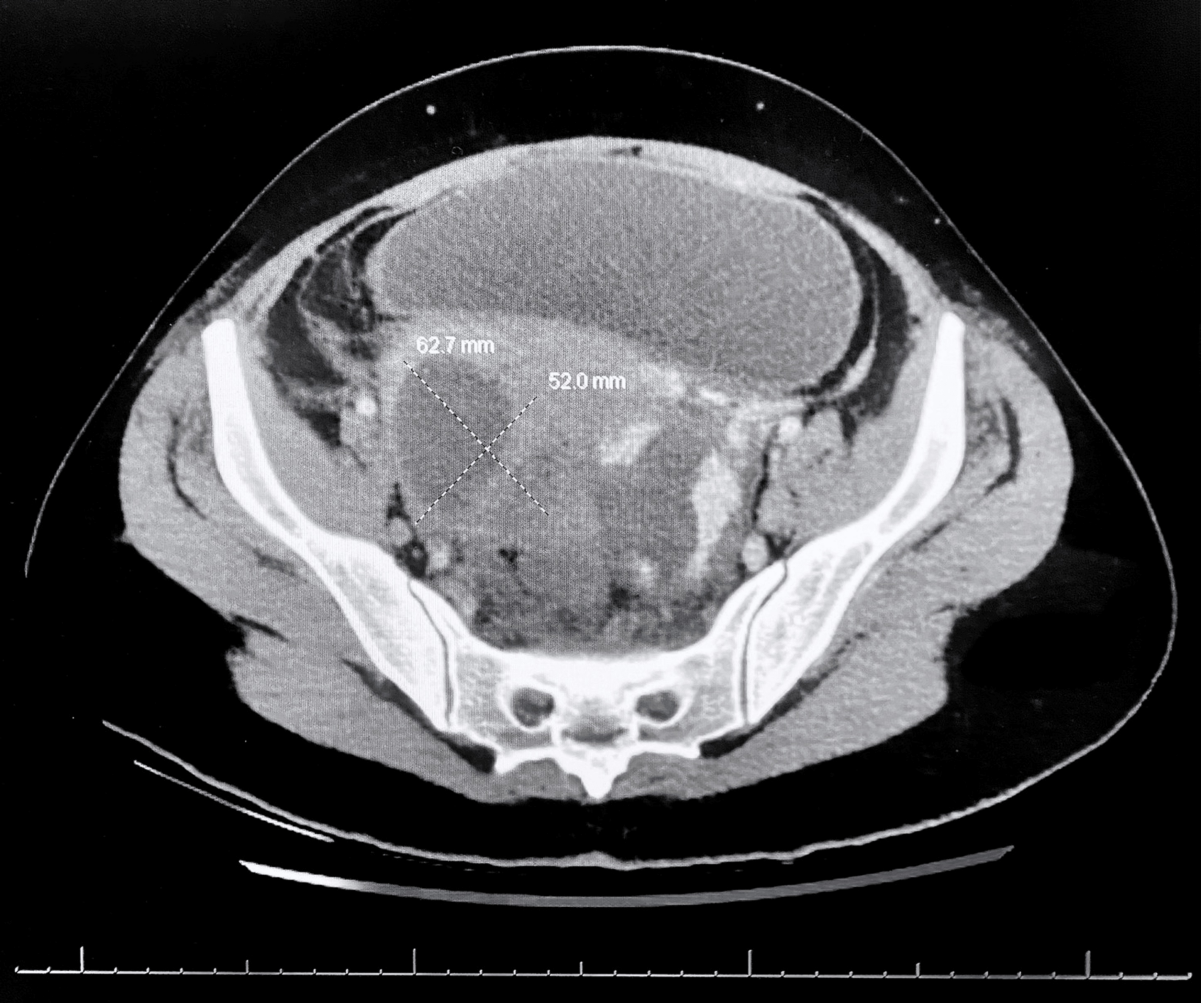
Axial contrast-enhanced CT scan of the pelvis revealing a large, irregular fluid collection measuring 62.7 mm x 52.0 mm, with adjacent inflammatory stranding, suggestive of a tubo-ovarian abscess and possible bowel perforation.
